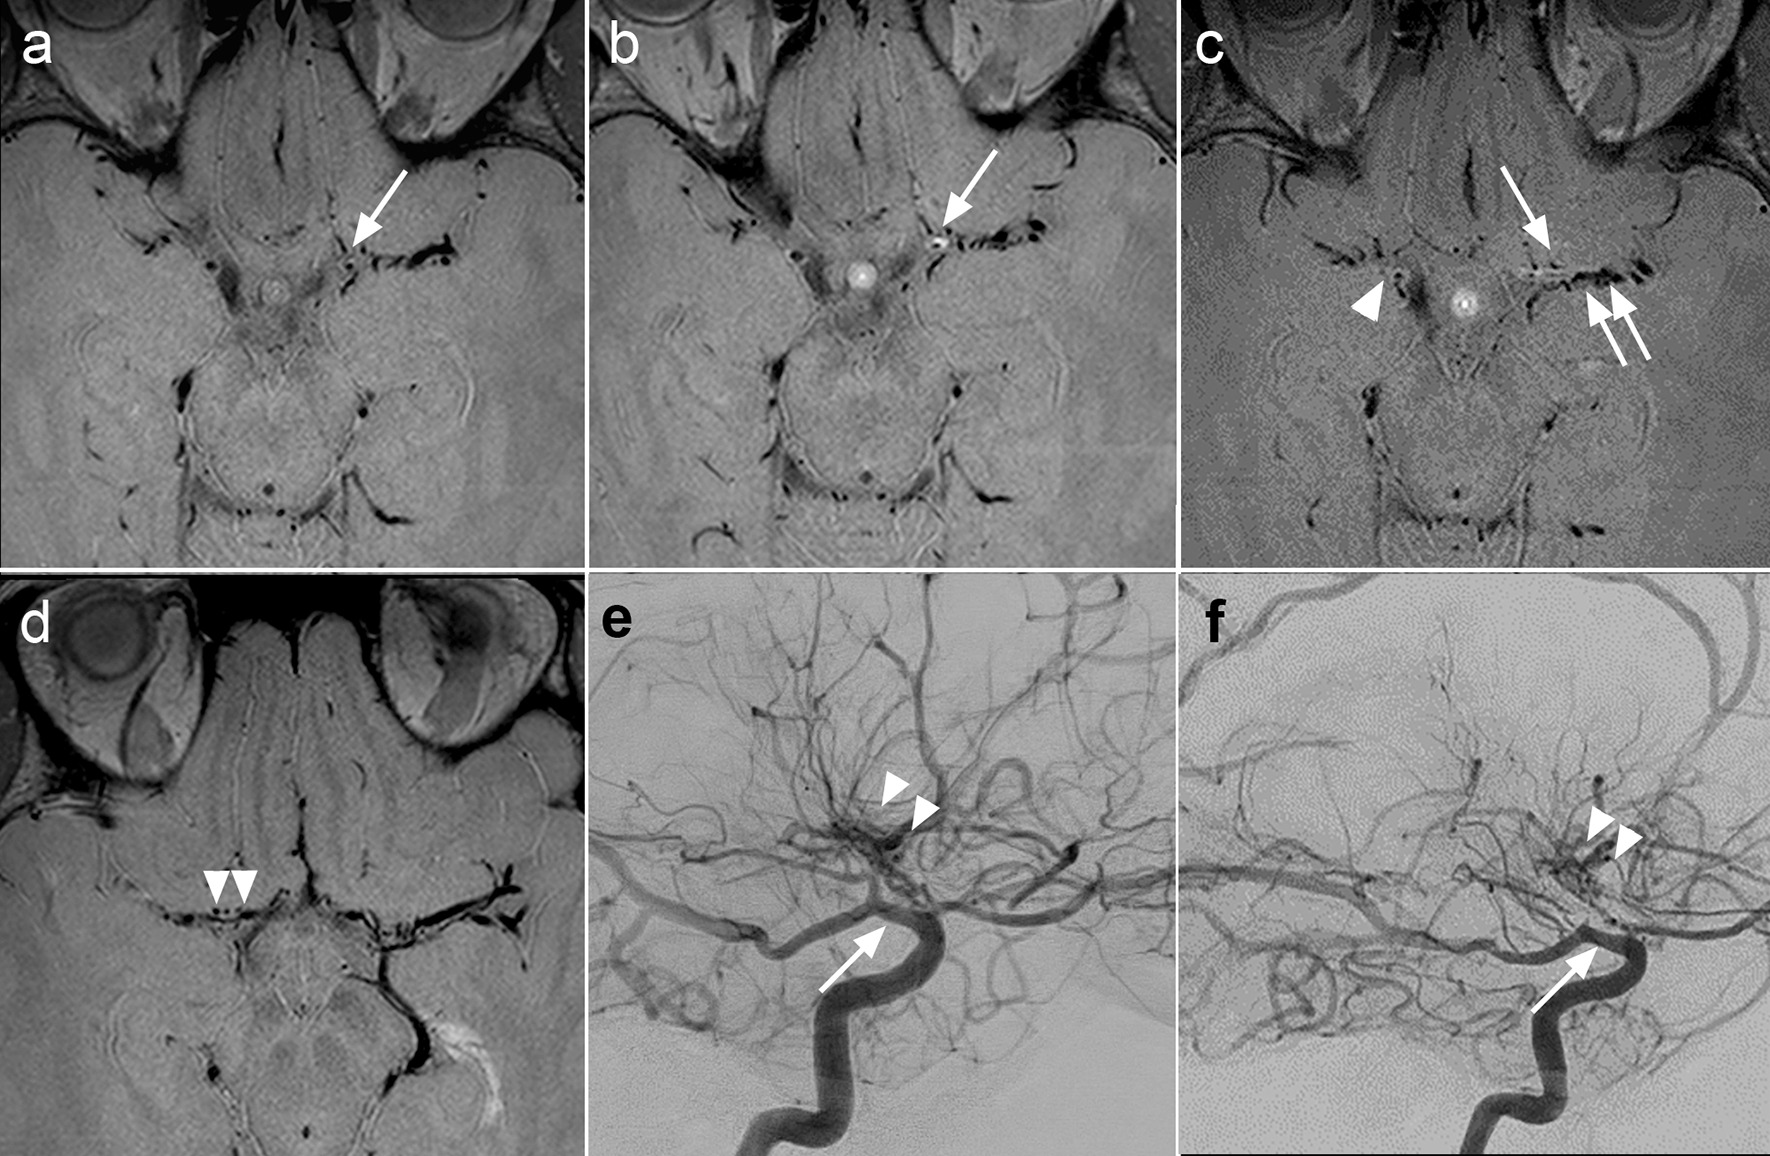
Eccentric grade II enhancement and absent enhancement of more distal involved segments. 31-year-old female with a history of bilateral deep border zone infarcts. The patient had a history of half-pack per day smoking for several years, but no other vascular risk factors. Trans-esophageal echocardiogram results were within normal limits. A comprehensive rheumatologic work-up did not reveal evidence of vasculitis or autoimmune disorder. CSF studies were unremarkable for evidence of vasculitis or active infection. Axial VWI image without gadolinium (a) demonstrates subtle eccentric thickening of the anterior wall of the left ICA terminus with negative remodeling (white arrow). Axial VWI with gadolinium (b) demonstrates eccentric grade II enhancement of the left ICA terminus corresponding to the area of eccentric thickening (white arrow). Axial VWI images with gadolinium at the level of the M1 segments (c, d) demonstrates faint (grade 1) circumferential wall enhancement of the distal right ICA (white arrowhead, c) proximal right M1 segment (white arrowheads, d), and proximal left M1 segment (white arrow, c). In distinction, vessel wall enhancement was absent in the mid to distal left M1 segment (double white arrows, c). The left middle cerebral vein is seen (double white arrowheads, f). Two-year follow-up right lateral (e) and left lateral (f) DSA images demonstrate progressive marked tapered stenosis of the bilateral ICA termini (white arrows), bilateral proximal A1 segments, and bilateral M1 segments as well as proliferation of lenticulostriate collaterals (white arrowheads), consistent with MMD
